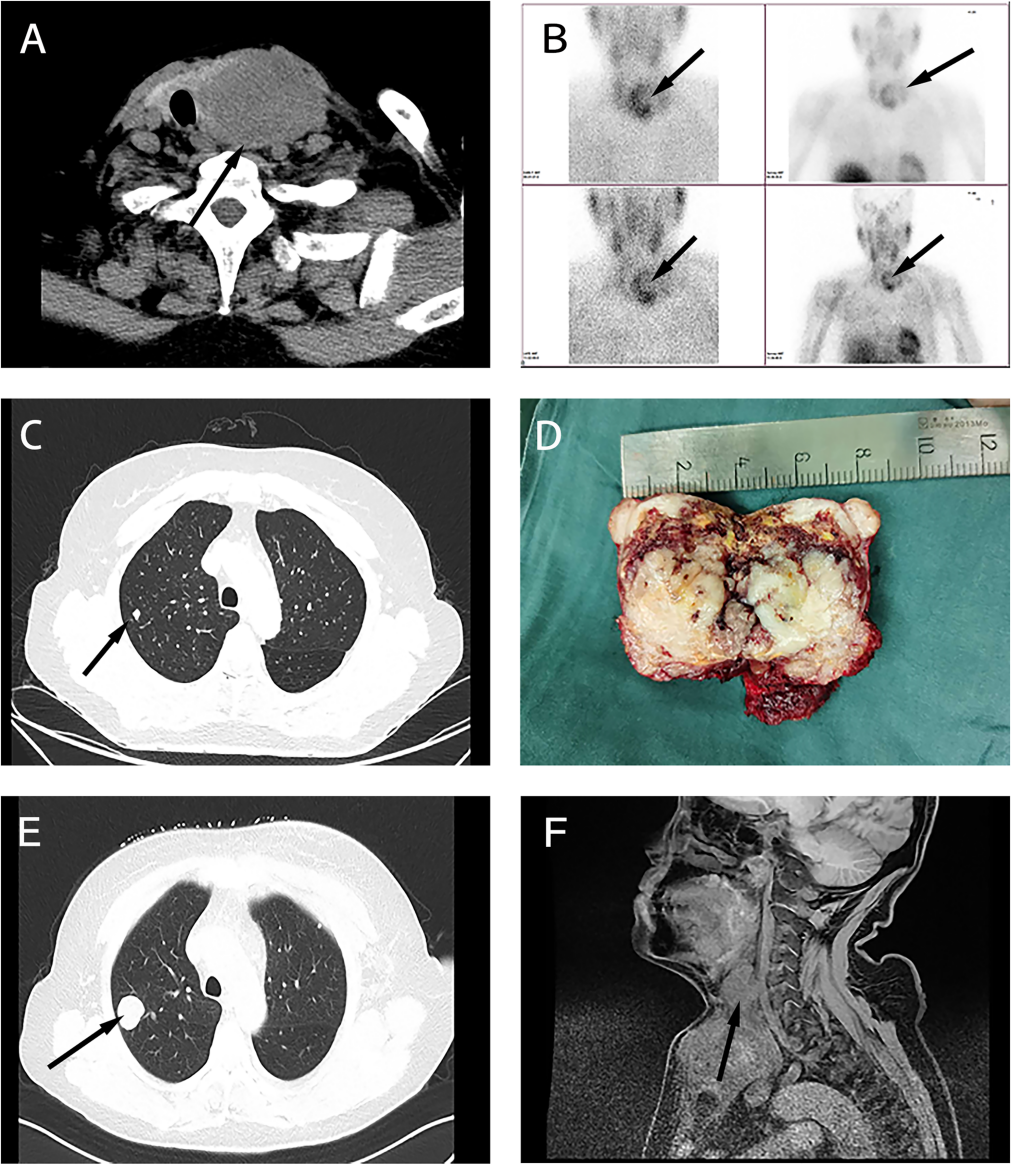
Clinical characteristics of the sarcomatoid parathyroid carcinoma (SaPC) case. (A) Neck CT showed the giant tumor. (B) Tc-99m sestamibi scintigraphy suggests abnormal nuclide uptake in the left thyroid. (C) Chest CT presented micro pulmonary nodule before surgery. (D) The profile of the resected tumor. (E) Chest CT shows pulmonary metastasis at the closely located position in preoperative chest CT (C). (F) Enhanced MRI showed extensive local organ and tissue invasion.
